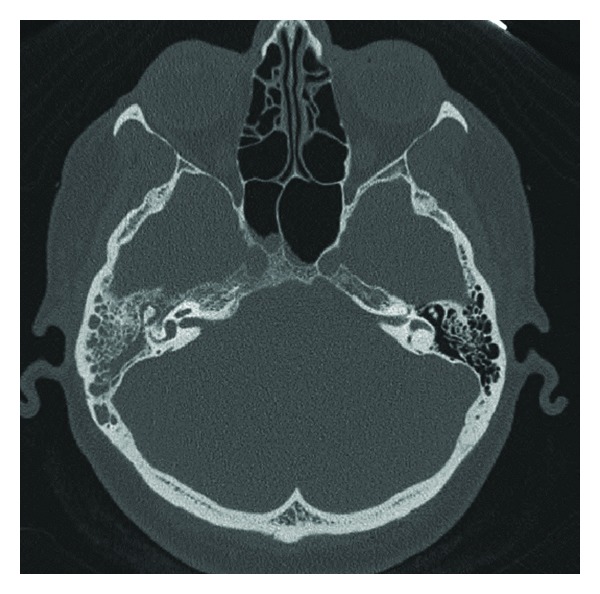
Axial high-resolution CT scan showing a right tympanomastoid homogenous opacity and petrous bone remodeling.
